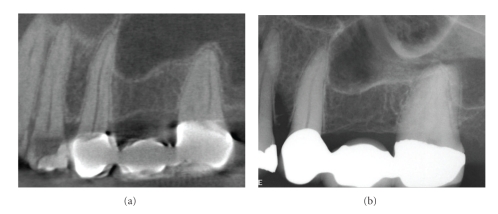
A female patient presented with a twenty-year history of mostly continuous, unilateral, poorly localized severe aching of the maxillary left quadrant. The pain was not associated with sensory loss or other physical signs and pulp tests, and conventional imaging studies were within normal limits. Clinically there was no cessation of pain after administration of local anesthetic. This neuropathic pain syndrome, initially termed atypical facial pain, is more recently known as persistent idiopathic facial pain (PIFP). PIFP refers to pain along the territory of the trigeminal nerve that does not fit the classic presentation of other cranial neuralgias. Diagnostically challenging, PIFP is frequently misdiagnosed and is often attributed by patients to dental procedures, facial trauma, and rarely, by some clinicians, as Neuralgia-Inducing Cavitational Osteonecrosis (NICO). Dynamic visualization of sequential curved planar parasagittal CBCT-reformatted images at 0.076 mm thickness (a) confirmed the absence of obvious pathosis of odontogenic origin as diagnosed from the original intraoral periapical of the region (b). Note the radiolucent area within the coronal portion of the first molar under the radiopaque disto-occlusal restorative material; this represents a streak artifact due to “photostarvation” in the horizontal plane due to the attenuation of adjacent amalgam and radiopaque material and subsequent reduction in available data for image reconstruction. A negative CBCT imaging finding is often very reassuring for these unfortunate patients who often question a nonodontogenic diagnosis. Psychiatric symptoms of depression and anxiety are prevalent in this population and compound the diagnostic conundrum.
